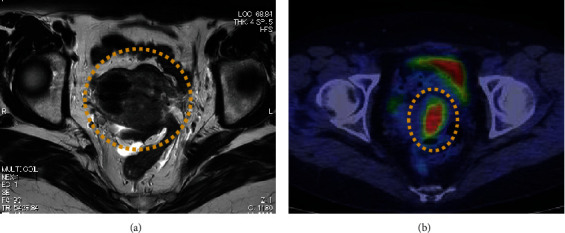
Imaging showing a tumor in the uterus 24 months after small bowel cancer surgery. (a) Contrast-enhanced computed tomography (CT) showing uterine enlargement. (b) Positron emission tomography (PET)/CT showing accumulation in the uterus.
